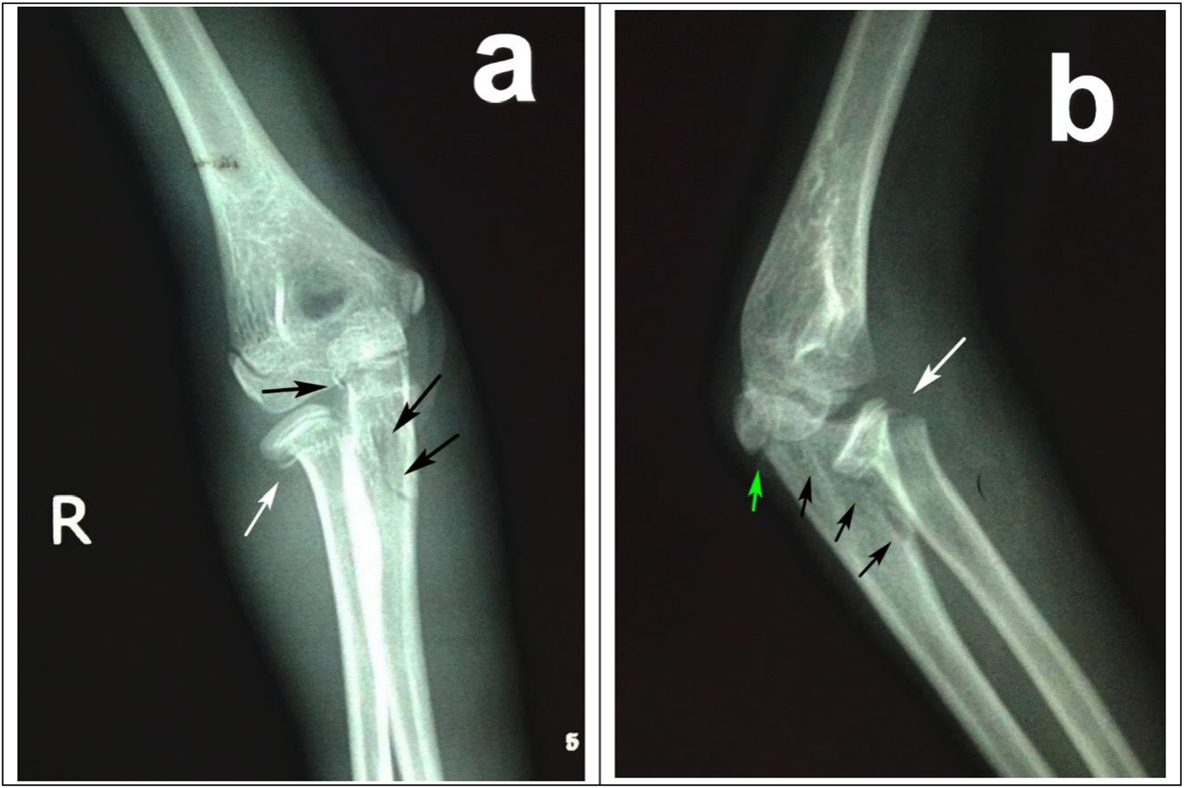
a Initial AP X-ray showed Jeffery type-1 radial neck fracture, indicating a lateral angulation of the radial head (White arrow) as a result of valgus stress, and proximal ulnar fracture (Black arrow). b Initial lateral X-ray showed anteriorly angulated radial neck fracture with intact radiocapitellar joint (White arrow) and proximal ulna fracture with fracture line going through olecranon epiphyseal plate (Green arrow) and potential anterior bowing (Black arrow)
